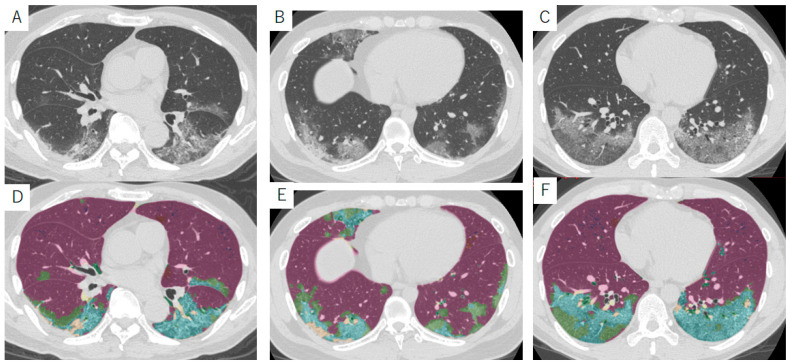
Typical CT images and their segmented images of patients with COVID-19 pneumonia. All segmentations were performed using QZIP-ILD. (A,D) Images from a 70-year-old man. The lesion area is 21% of the lung area in this slice. (B,E) Images from a 28-year-old man. The lesion area in this slice is 25% of the lung area in this slice. (C,F) Images from a 39-year-old man. The lesion area in this slice is 30% of the lung area in this slice. All patients were admitted and required oxygen therapy. These figures were used in Kanagawa Prefecture, Japan, during the COVID-19 pandemic as a reference to determine the need for hospital admission. Abbreviations: COVID-19, coronavirus disease 2019.
